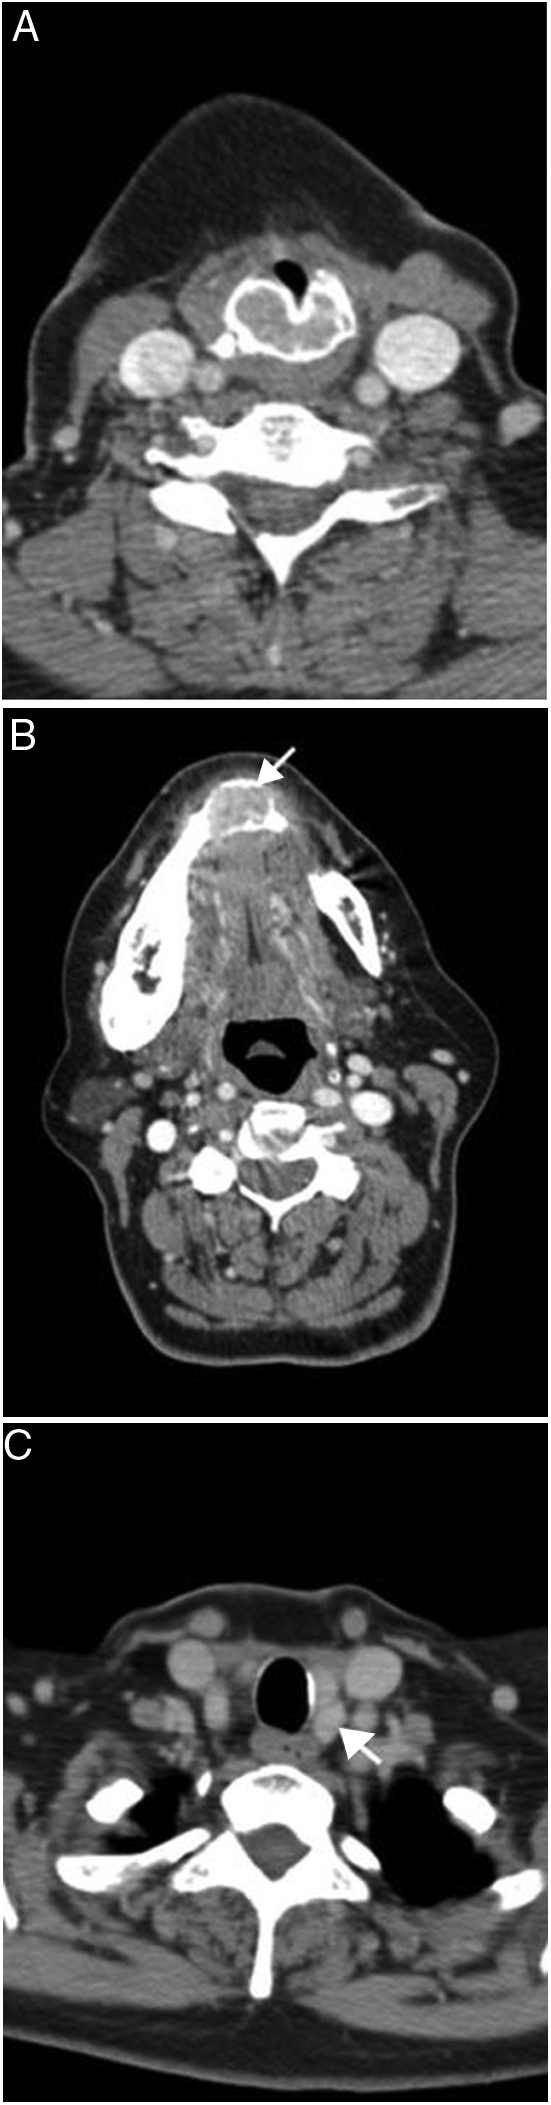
(A–C) 52 year old woman with primary hyperparathyroidism and multiple brown tumors. Contrast enhanced CT image (A) shows expansile mass-brown tumor arising from the cricoid cartilage. Mandibular lytic expansile mass is seen on more superior level CT image (B). Parathyroid adenoma is seen at left inferior parathyroid gland location (C).
